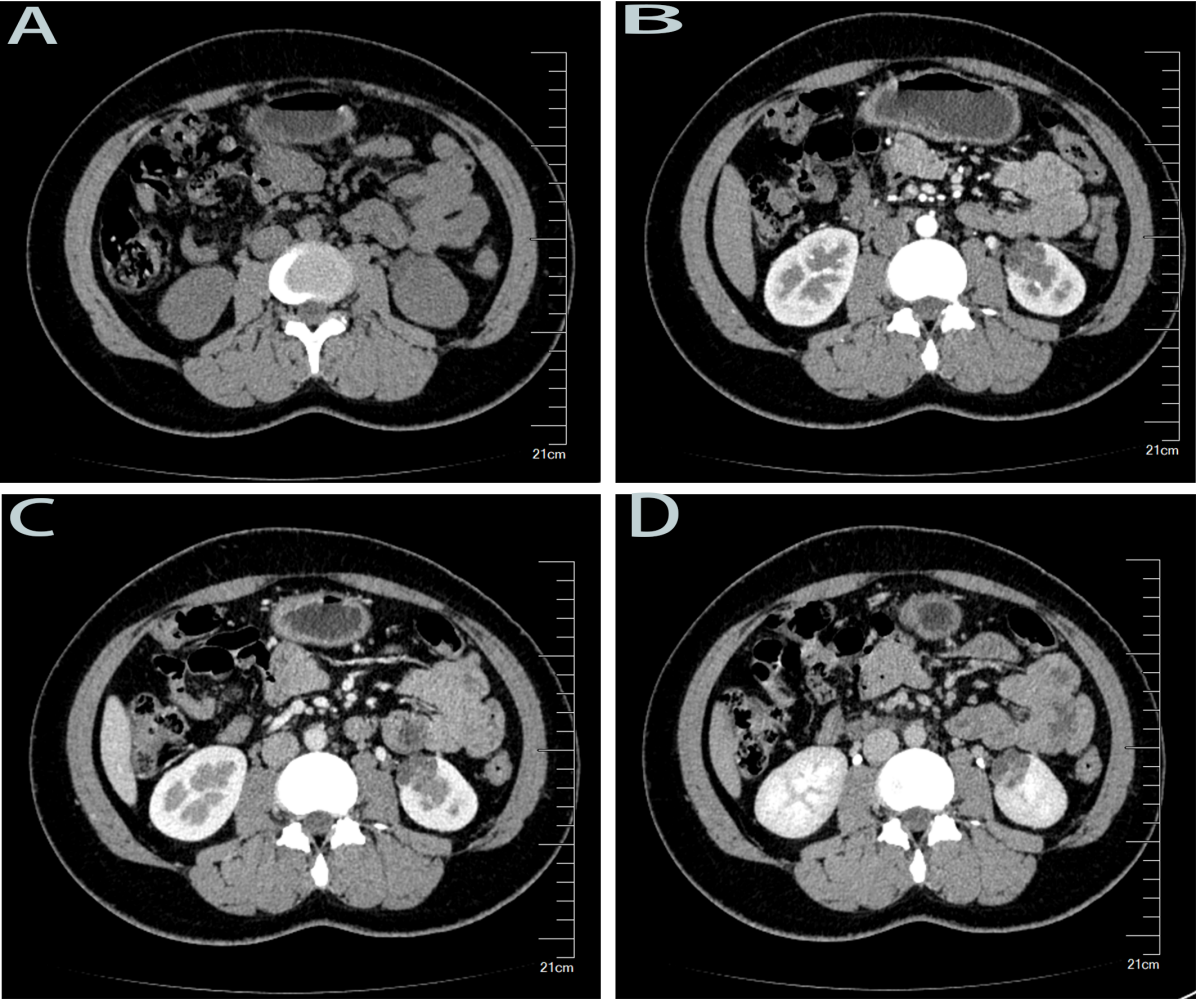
The CT imaging findings (non-contrast and contrast-enhanced scans of the whole abdomen) for this case upon admission are as follows: (A) Non-contrast CT scan: A cystic, slightly hypodense shadow is observed in the left kidney. (B) Contrast-enhanced CT scan, arterial phase: Mild to moderate enhancement of the cystic wall and septa within the mass is visible. (C) Contrast-enhanced CT scan, venous phase: The mass appears lobulated and contains multiple internal septations. (D) Contrast-enhanced CT scan, excretory phase: Patchy high-density shadows are noted in the left renal sinus.
